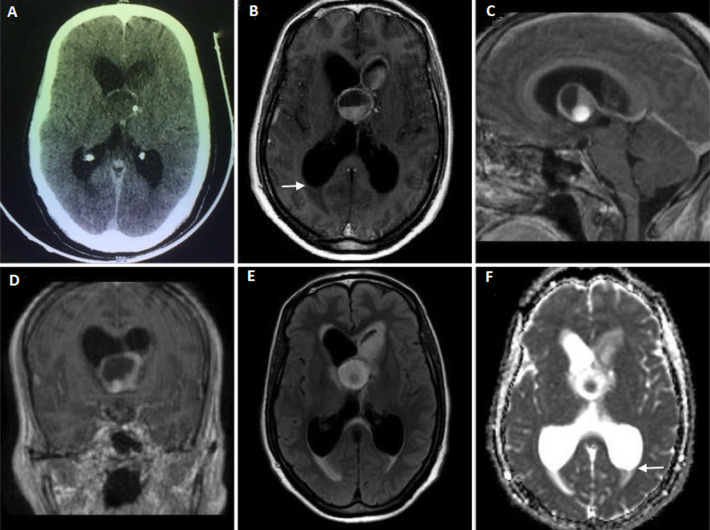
contrasted head CT scan; A) showing an isodense mass within the third ventricle with mild rim contrast enhancement and spot calcification. Contrasted axial; B) sagittal C) coronal; D) T1-weighted MR images showing a bilobate cystic lesion encompassing the third ventricle and the left frontal horn with fluid-fluid level and mild rim contrast enhancement; this lesion contains both hypointense and hyperintense components in FLAIR; E) and in apparent diffusion coefficient map; F) note the debris in the occipital horns of the lateral ventricles with a fluid-fluid level (white arrow)
